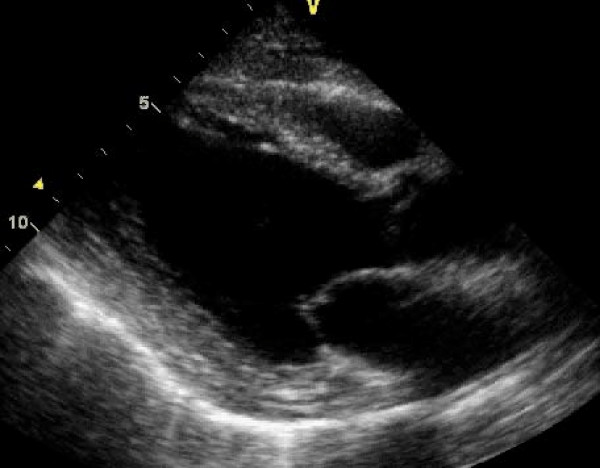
A transthoracic echocardiogram parasternal long axis view demonstrating a mildly dilated left ventricle and global LV systolic dysfunction.
